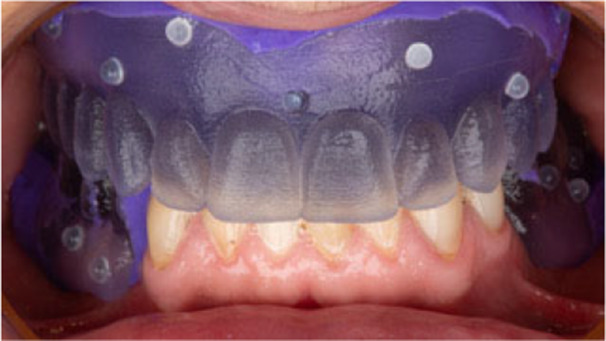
Radiographic template designed in a case of a fully edentulous patients with inadequate existing dentures.
